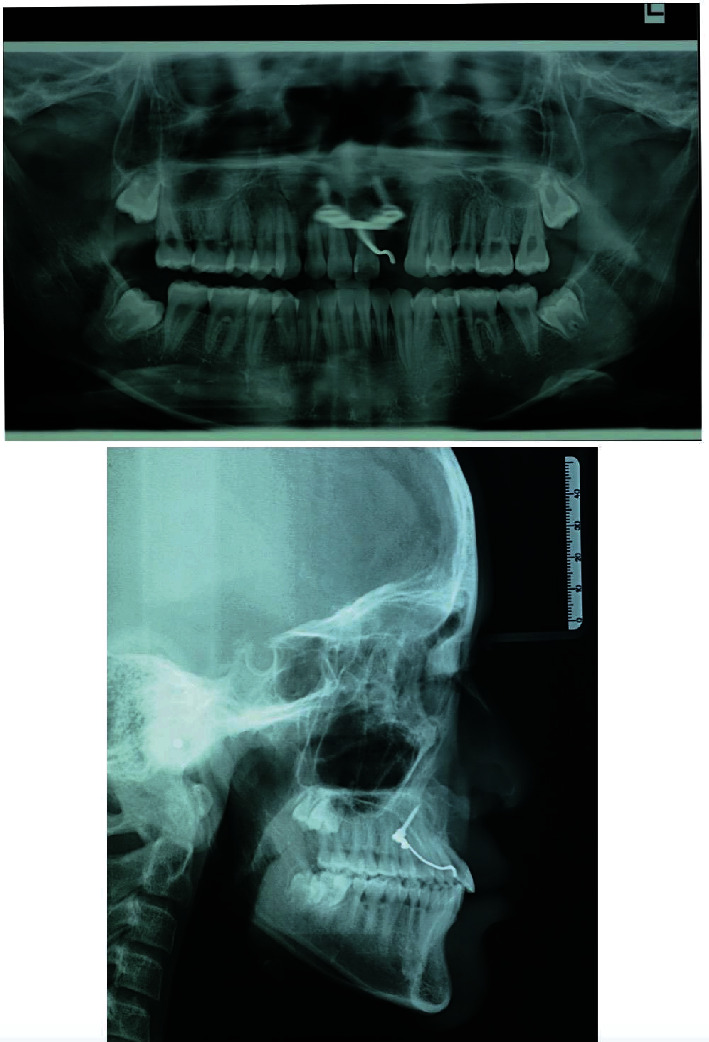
Final panoramic radiograph and lateral cephalogram of the skull.
